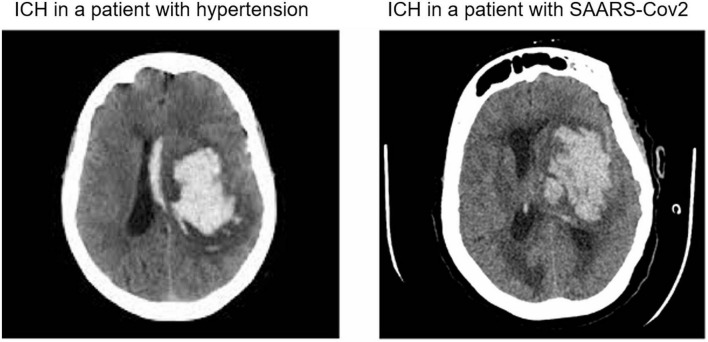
Representative computed tomography (CT) scans of patients with intracranial hemorrhage (ICH) suffering from hypertension or SARS-CoV2 infection/COVID-19.
